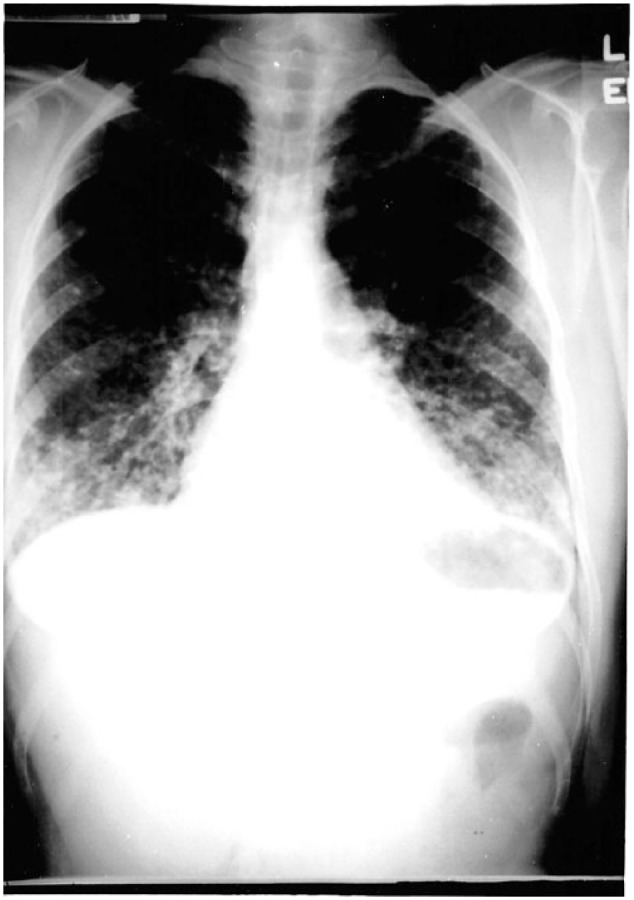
1st Chest radiograph (Outpatient).
